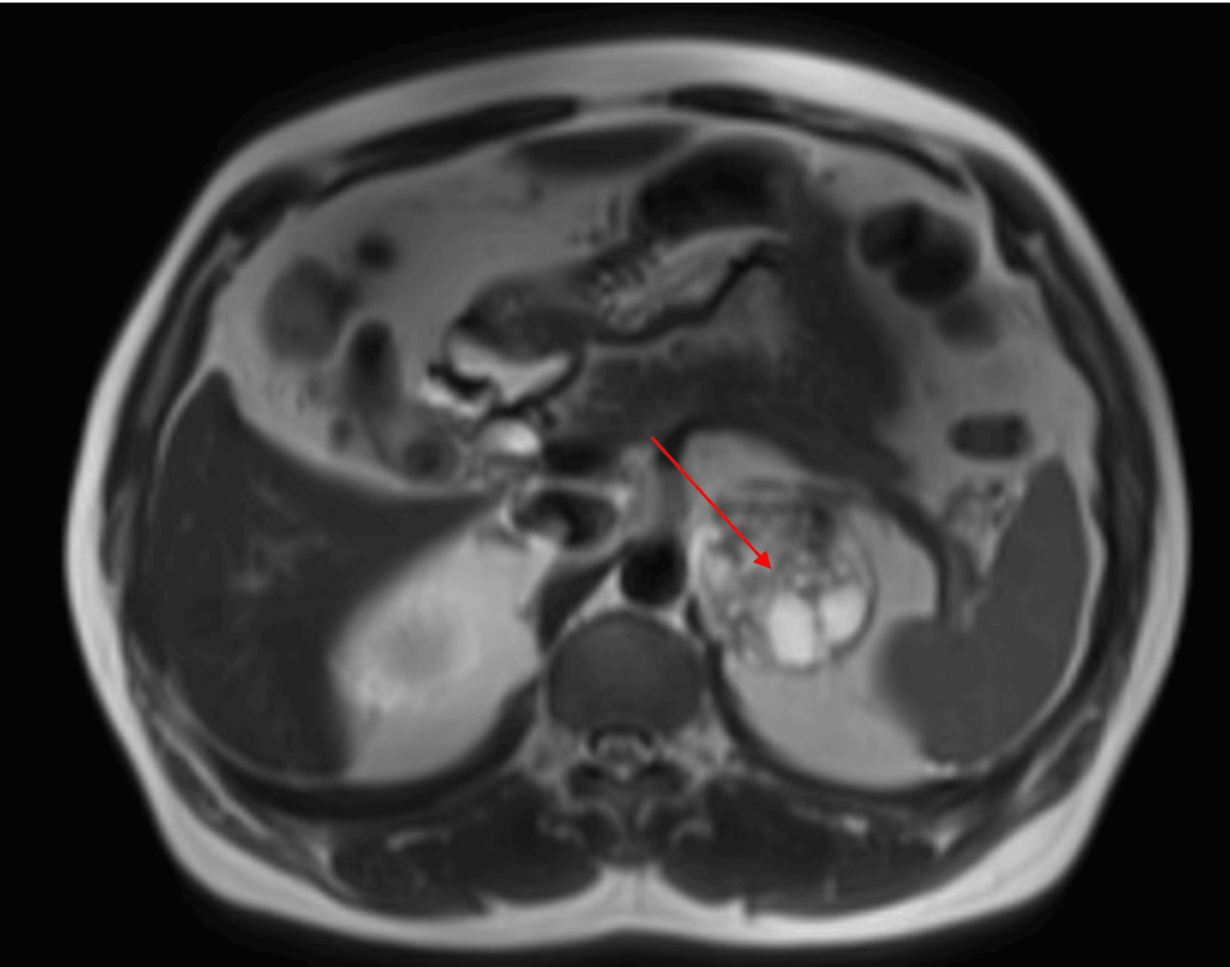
Axial abdominal MRI demonstrating a heterogeneous left adrenal mass (arrow) measuring 5.8 × 5.1 × 5.8 cm.
